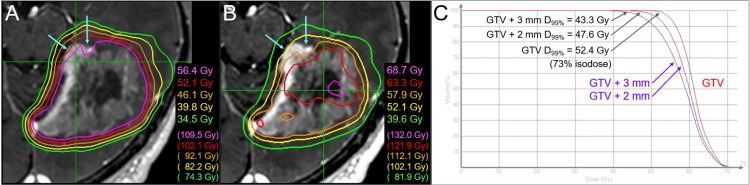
Dose distributions and DVHs for the upfront 15-fraction stereotactic radiosurgery The images show (A, B) representative isodose distributions superimposed onto axial contrast-enhanced T1-weighted image and (C) DVHs. (A, B) Nine representative isodose lines are shown to characterize the dose gradients mainly outside (A) and inside (B) the gross tumor boundary, along with the relevant BEDs based on the linear-quadratic formula with an alpha/beta ratio of 10 (BED_10_). The 52.1 Gy isodoses are shown in both, although the colors are different. The sufficient GTV coverages with 52.1-57.9 Gy are compromised near the brainstem (arrows in A, B). (C) The BED_10_s of 43.3 Gy, 47.6 Gy, and 52.4 Gy are 55.8 Gy, 62.7 Gy, and 70.7 Gy, respectively. The 52.4 Gy isodose is 73% relative to the maximum dose of the GTV. DVH: dose-volume histogram; BED: biologically effective dose; GTV: gross tumor volume; GTV + 2/3 mm: a structure generated by adding an isotropic 2/3 mm margin to a GTV boundary; D_99%_: a minimum dose encompassing at least 99% of the target volume
